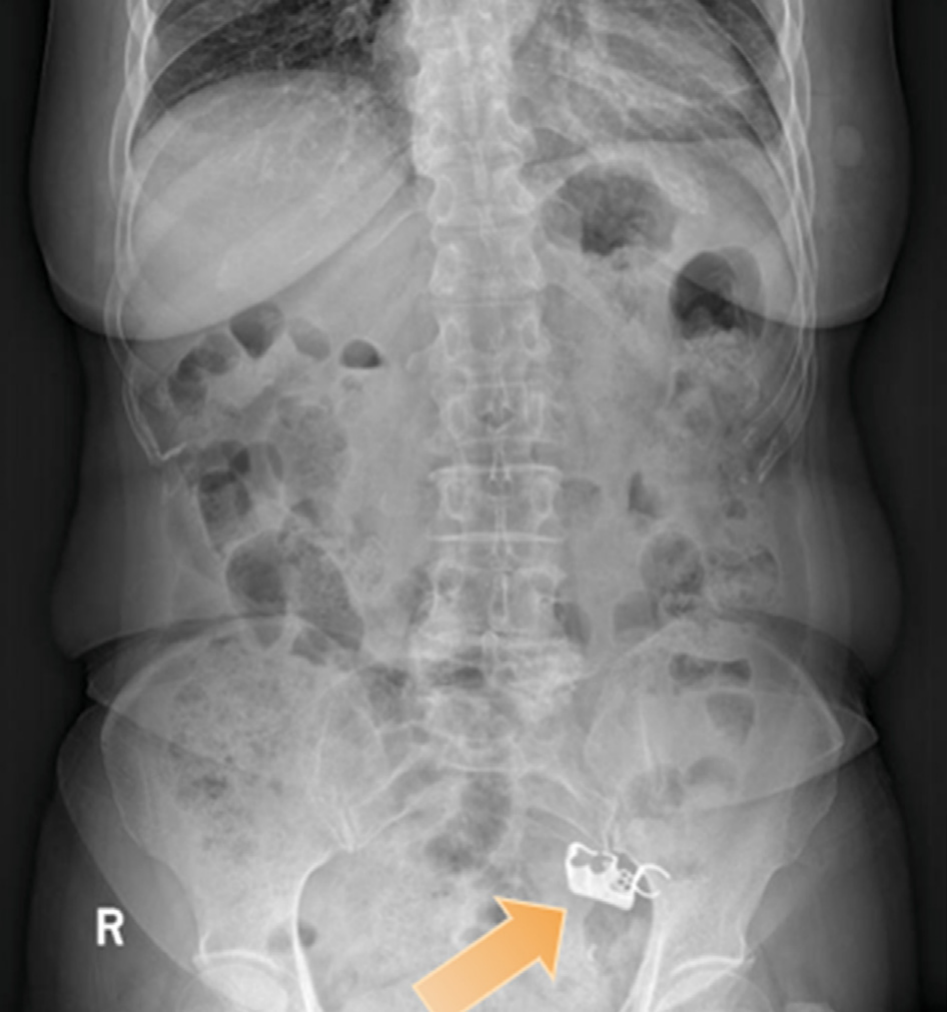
Abdominal radiograph showed a high-density shadow in the left lower abdomen.
